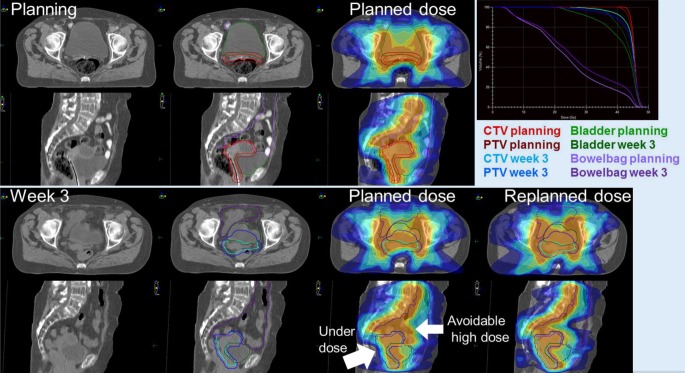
Example of how organ position can change as a function of different bladder and rectum filling. Planning computed tomography image and planned dose with filled bladder and rectum (*upper panel*); rescanning at week 3 (*lower panel*) with less filled bladder. Planned dose and recalculated dose distribution overlaid in color wash; *white arrows* depict underdose and avoidable high dose that were corrected by choosing a better suitable plan from the library (*lower panel*, re-planned dose). *CTV* clinical target volume, *PTV* planning target volume
